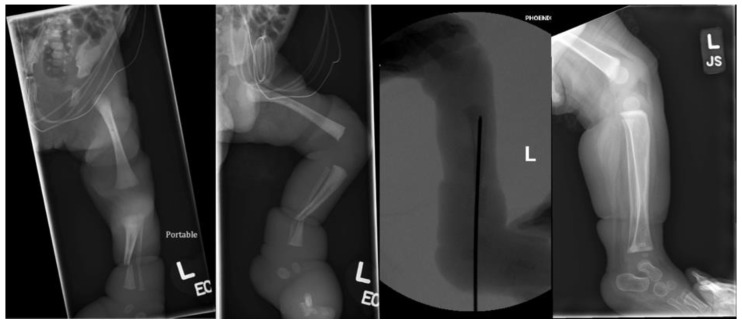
Radiographs of Patient 3: preoperative AP view, preoperative lateral view, intraoperative lateral view, and 1-year postoperative lateral view (left to right).
